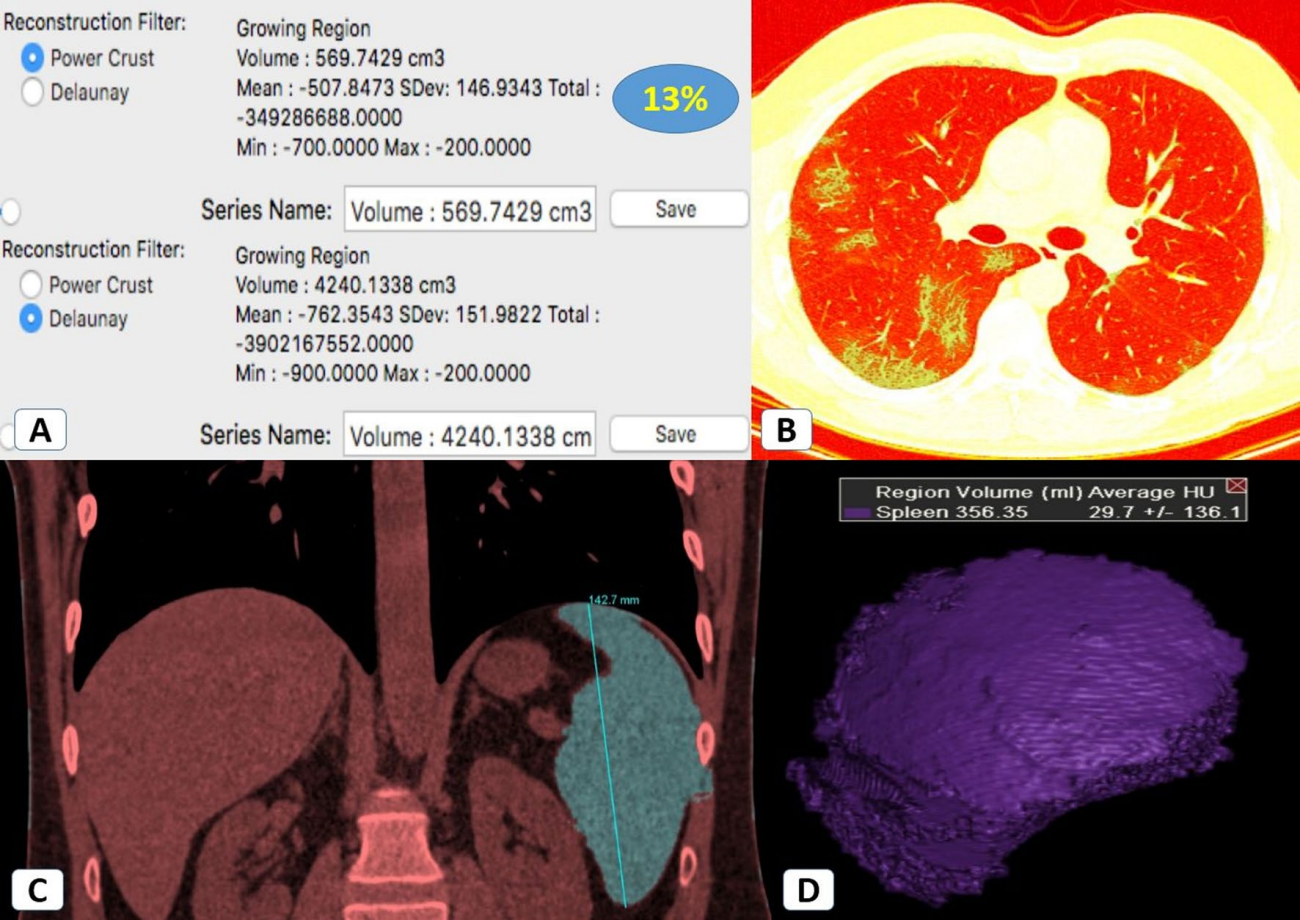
Splenomegaly in a 33-year-old male patient with mild COVID-19 infection: A, B Volumetric CT assessment of the lung revealed 13% pathological lung involvement. C Coronal CT (mediastinal window) showed an increased craniocaudal bi-polar diameter of the spleen (14.2 cm). D CT volumetry of the spleen was 356 cc
